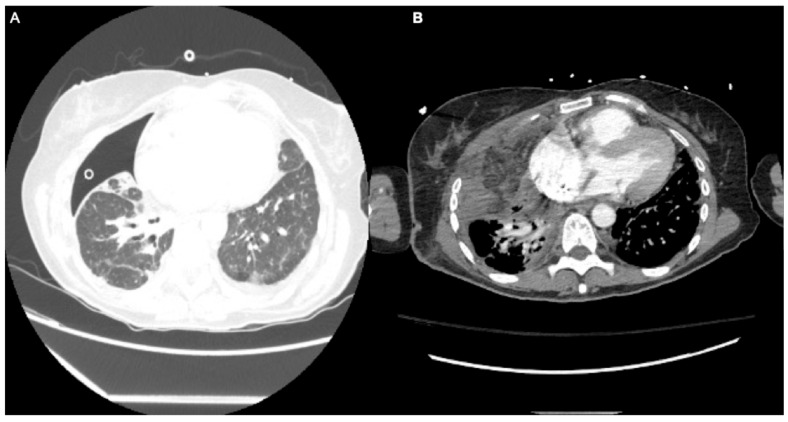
Patient B, (A)—CT chest with chronic interstitial lung disease, chronic parenchymal opacities, and chronic scarring throughout both lungs. (B)—Postoperative CT scan with omental flap in place.
